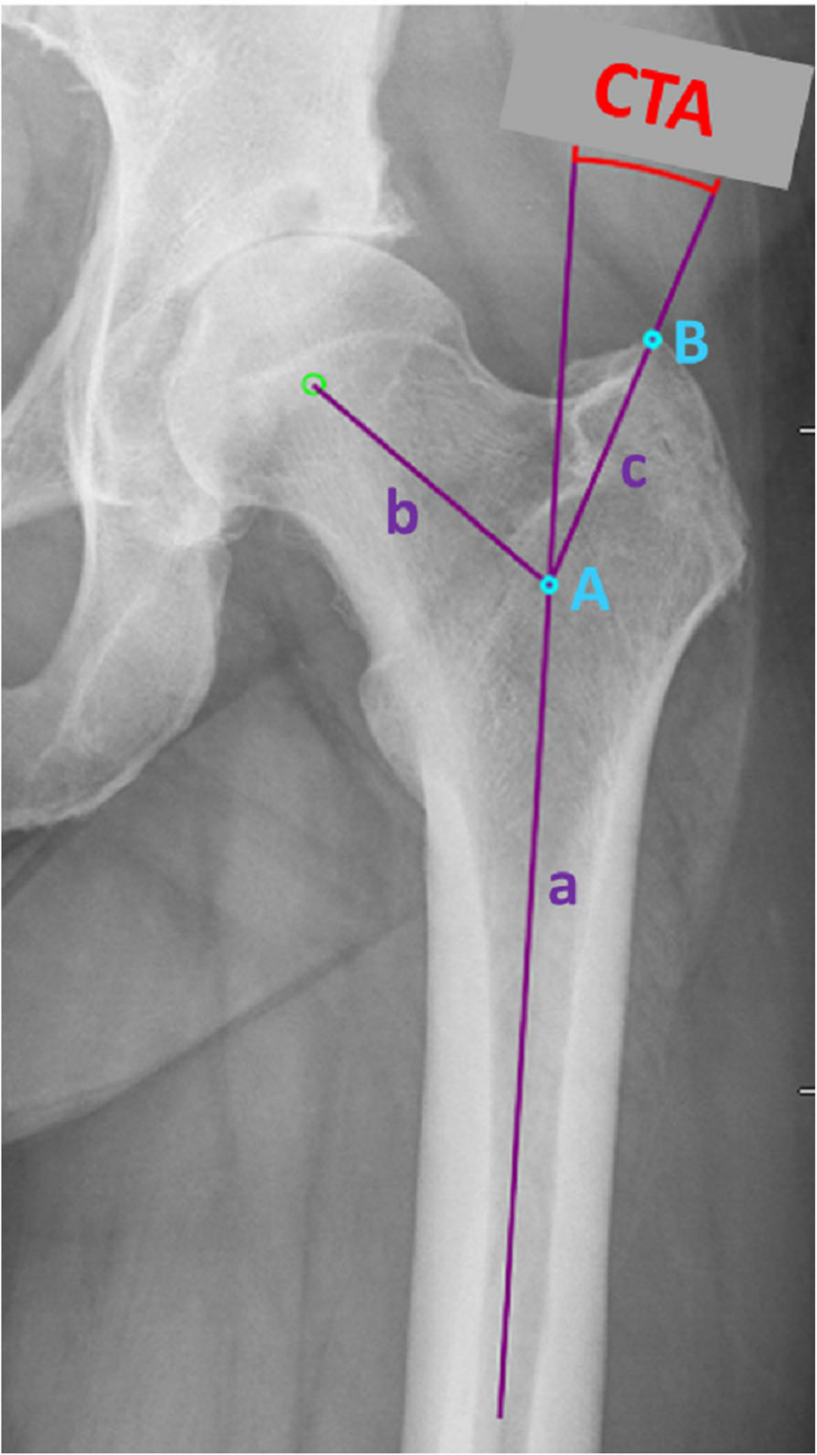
Measurement of the critical trochanter angle (CTA). The angle crest (A) is defined as the intersection of the femoral shaft axis (a) and the femoral neck axis (b). A leg (c) between the angle crest (A) and trochanter vertex (B) is build, and the CTA is measured between this leg and the femoral shaft axis
